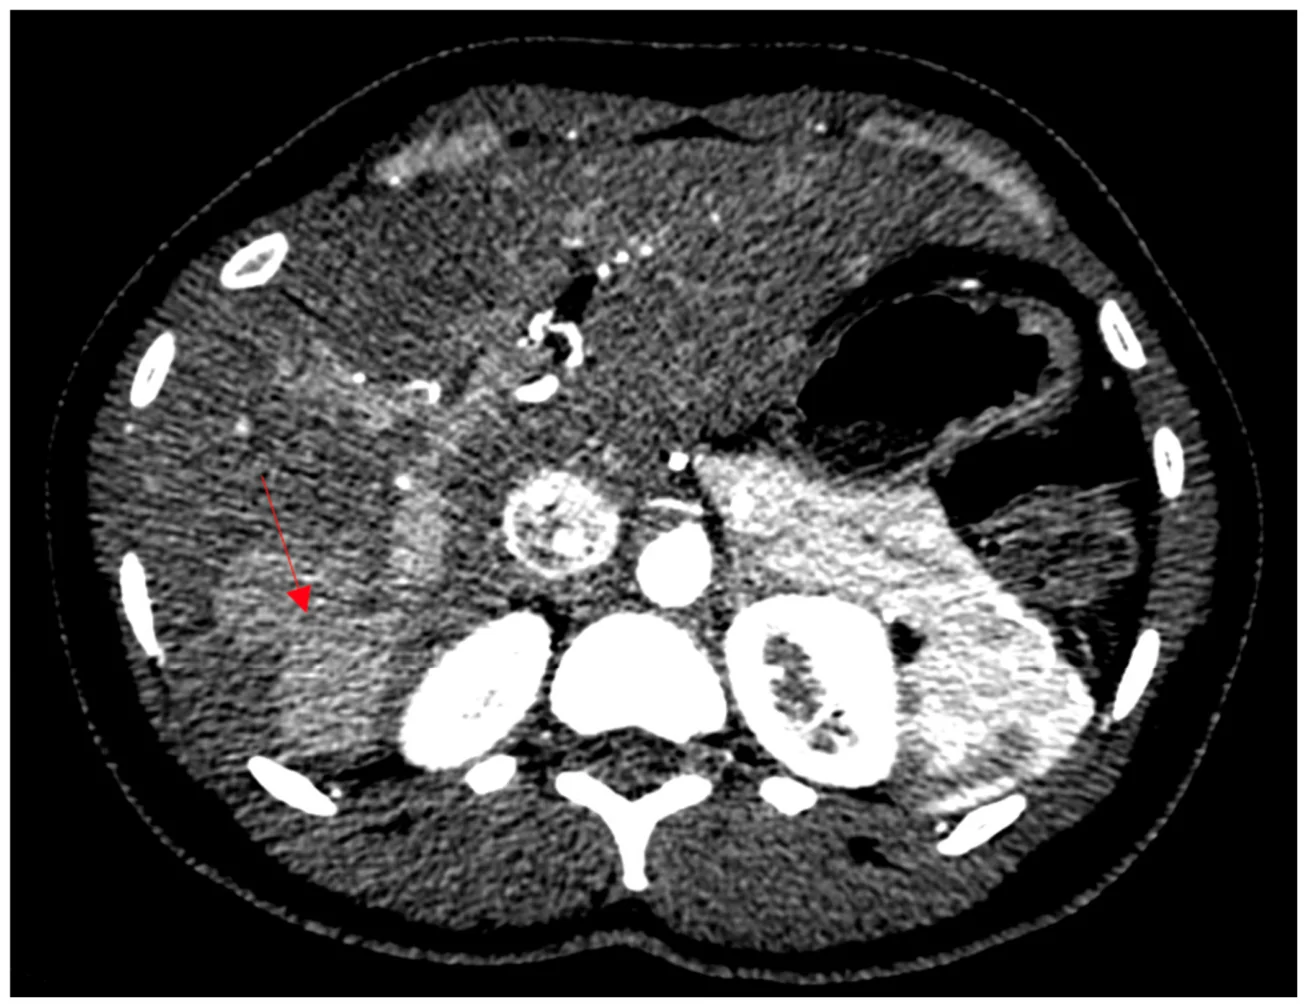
Axial CT showing presence of intrahepatic portosystemic venous shunt (arrow) between the posterior branch of the right portal vein and the right hepatic vein.
